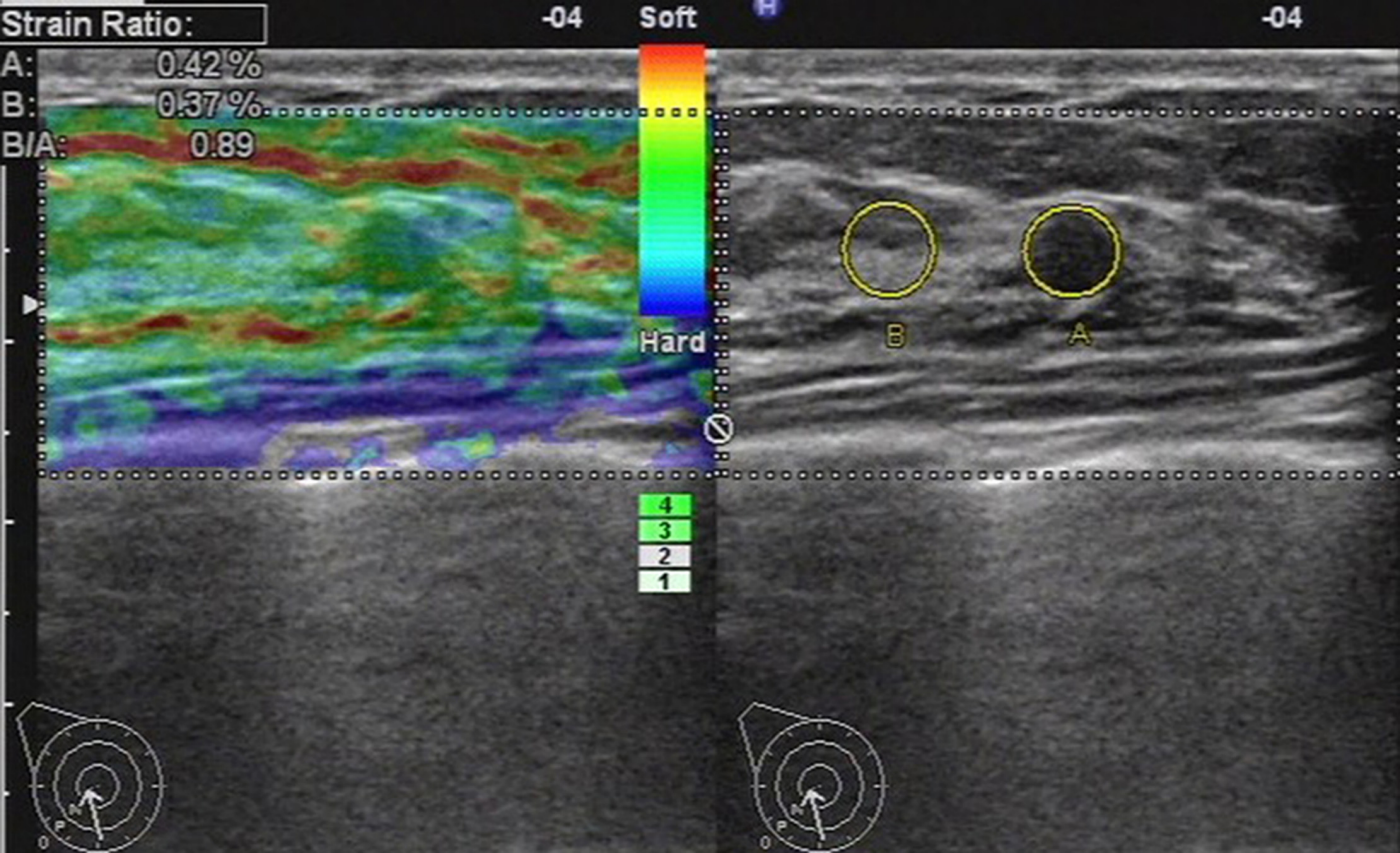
Ultrasonic elastographic image of a DCIS 32-year-old woman. The lesion was scored 1(benign) with the 5-point scoring method. The strain ratio was 0.89(benign) with the strain ratio method at the same breast tissue depth as the reference.
